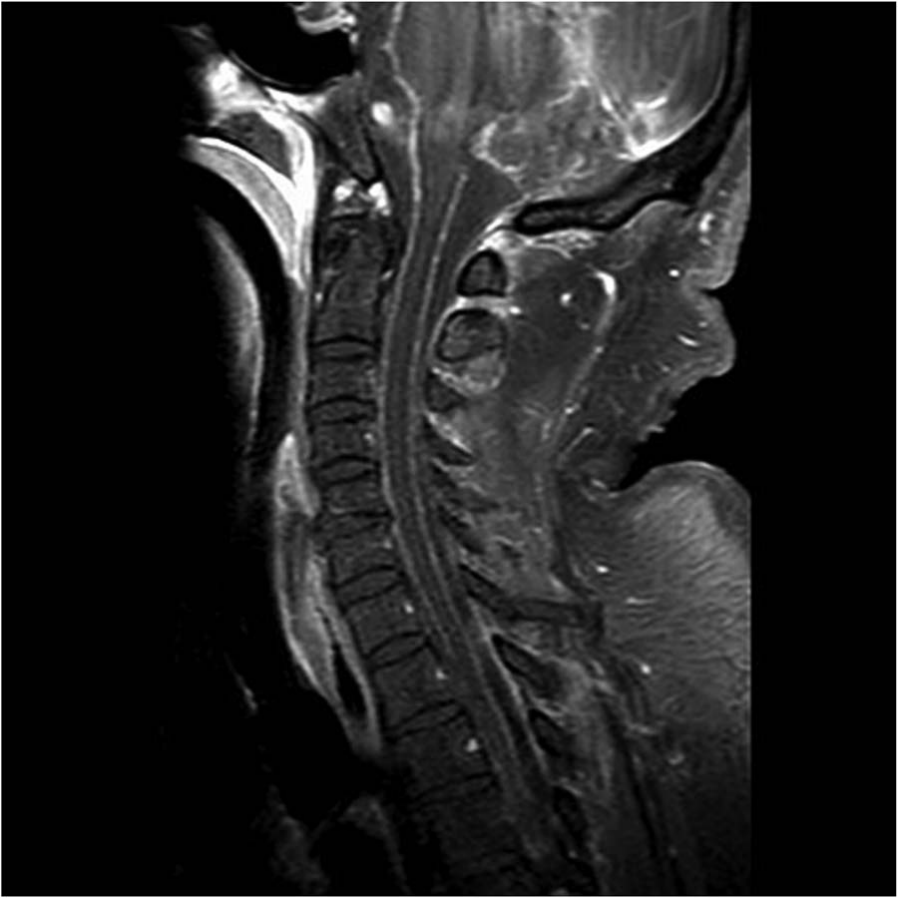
MRI of the spinal cord revealing leptomeningeal enhancement along the cervical and thoracic spinal cord and cauda equina nerve roots.
